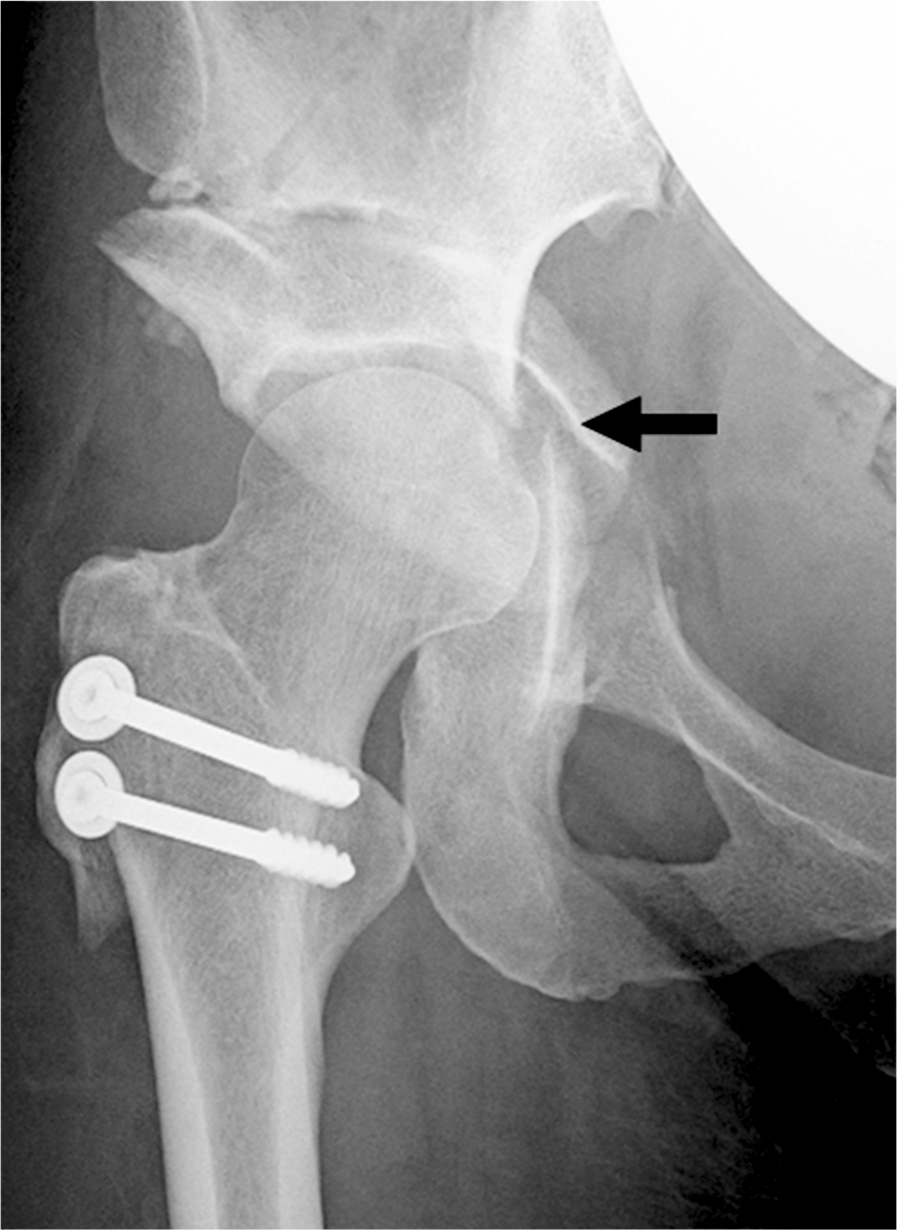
A radiograph of the same patient made 4 weeks after osteotomy. The patient reported right buttock pain 3.5 weeks postoperatively. A fracture of the posterior column (arrow) was revealed, which was not seen in Figure 1.
